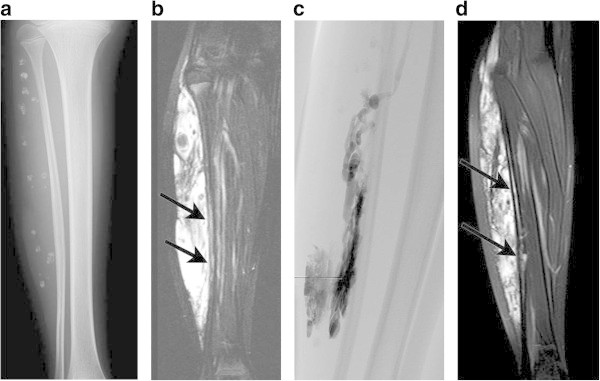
A 12-year-old-female with symptoms of pain and swelling of the right calf. A. Radiograph of the right calf demonstrates phleboliths and cortical irregularity in the fibula. B. The coronal fat-saturated T2-weighted MR image before treatment shows a large and infiltrating mass adjacent to the fibula with diffuse marrow signal abnormality (arrows). C. Direct puncture phlebography reveals VM with dysmorphic veins and early venous return of the peroneal vein (Type 3). After sclerotherapy with ethanol, the patient had onset of temporary peroneal nerve paralysis. D. Although the lesion shows a decrease in thickness and signal intensity on MRI, the marrow signal abnormality (arrows) remains unchanged. She reported no change in symptoms and indicated dissatisfaction on the questionnaire.
